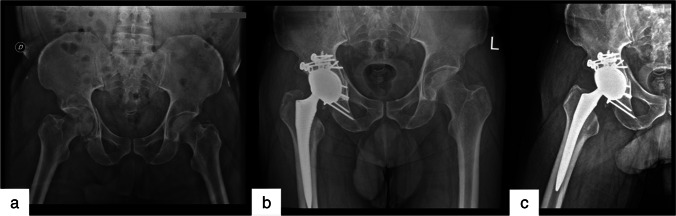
Pelvic x-rays of a 64-year-old man with transverse + posterior wall fracture of the right acetabulum (a). CT scan revealed negative prognostic factors (posterior wall comminution, marginal impaction, femoral head impactions). CHP was performed restoring the overall shape of the acetabulum with double plating and implanting a multihole revision acetabular component (b, c)
